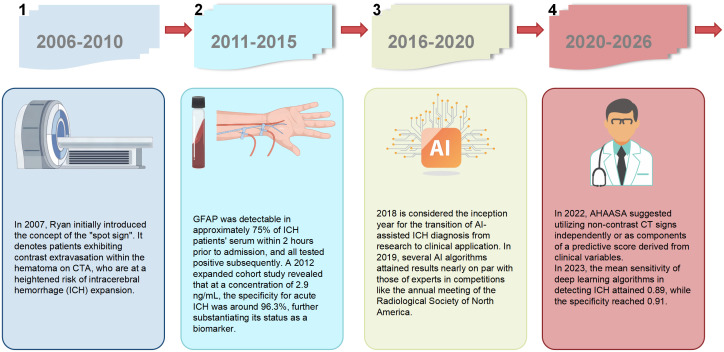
Advances in diagnostic techniques for ICH. In 2007, the “spot sign” was initially proposed as a predictor for the risk of HE. In 2010, the AHA/ASA guidelines were revised to endorse CTA and contrast-enhanced CT for assessing the risk of HE (Class Ilb, Level B evidence). Serum GFAP can be detected in roughly 75% of patients with ICH within 2 hours prior to admission and subsequently in nearly all patients, owing to the abrupt disruption of the BBB and rapid damage to astrocytes induced by acute ICH. Al-driven imaging interpretation technology assumes a pivotal role in stroke diagnosis, facilitating automated ICH detection and differentiation of hemorrhage subtypes. Furthermore, it possesses the capability to identify skull fractures, midline shift, and the mass effect of hematomas. AHA, American Heart Association; Al, artificial intelligence; ASA, American Stroke Association; BBB, blood-brain barrier; CT, computerized tomography; CTA, CT angiography; GFAP, glial fibrillary acidic protein; HE, hematoma expansion; ICH, intracerebral hemorrhage
